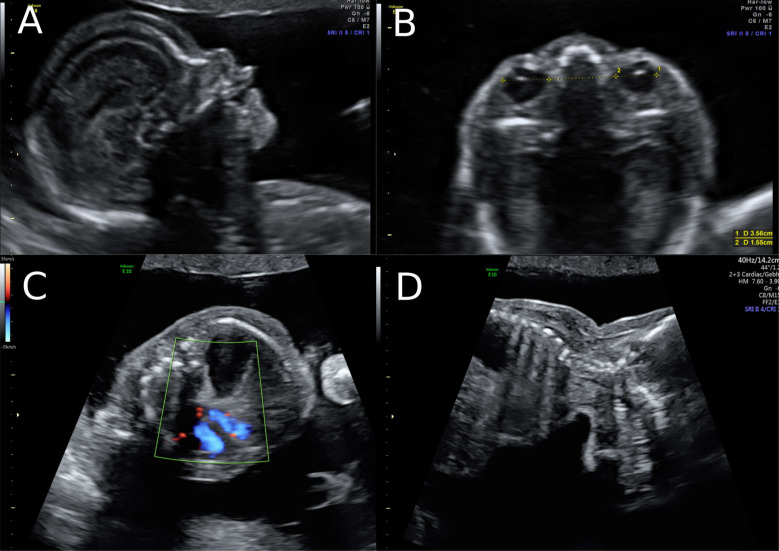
Ultrasound at 22 weeks of gestation (A,B) Striking profile and brain structure in fetus with Simpson-Golabi-Behmel syndrome. (C) Suspicion of congenital diaphragmatic hernia at 22 weeks of gestation and (D) increased subcutaneous fat tissue.
